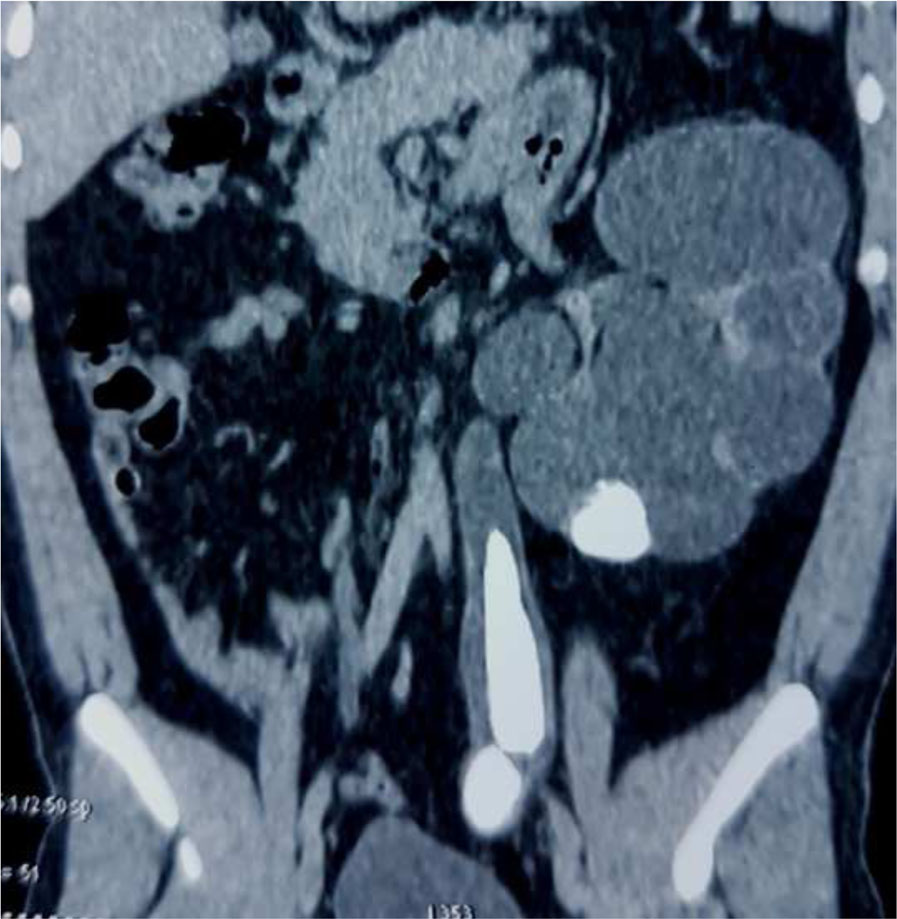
Coronal enhanced computed tomography urography showing severe hydroureteronephrosis associated with two giant left ureteral stones and a left inferior calyceal stone
